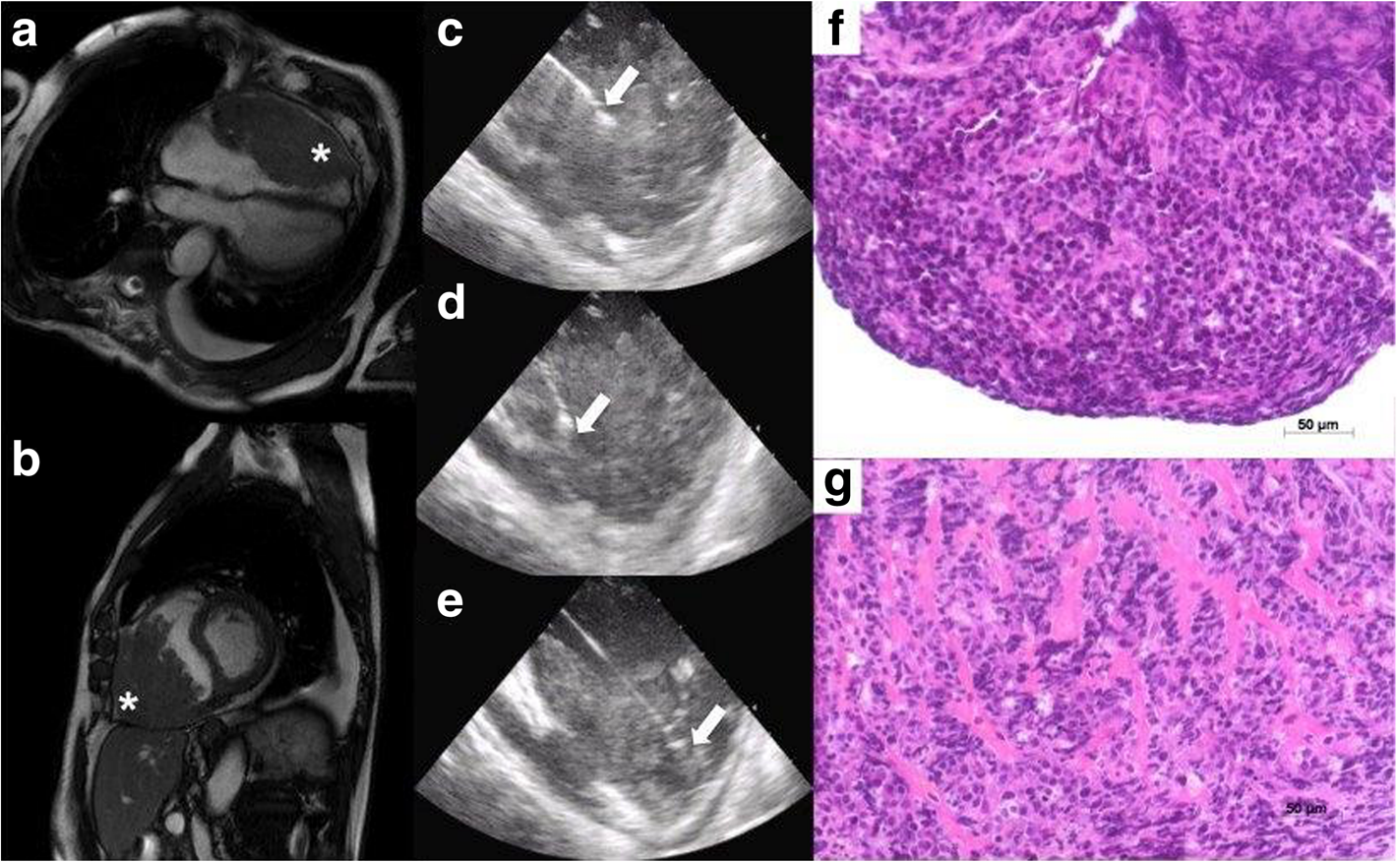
Cardiac magnetic resonance imaging: an irregular voluminous mass (12 × 7.6 cm, thickness of 5.5 cm) extending along the free wall of the RV to the tricuspid annulus is showed in panel (a and b) (white star). ICE was extremely helpful to identify the heterogeneity of the mass and to selectively place the bioptome, panel (c, d and e) (white arrow). Hystopathological diagnosis revealed a diffuse large B cell non-Hodgkin lymphoma, panel (f and g)
